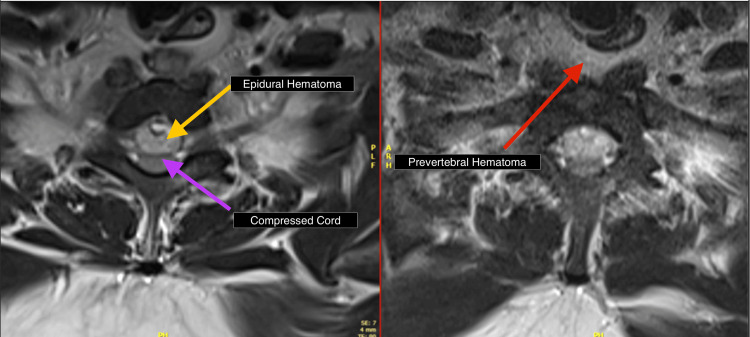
MRI Axial T2WI images reveal large prevertebral and intraspinal epidural hematoma with spinal cord compression.
